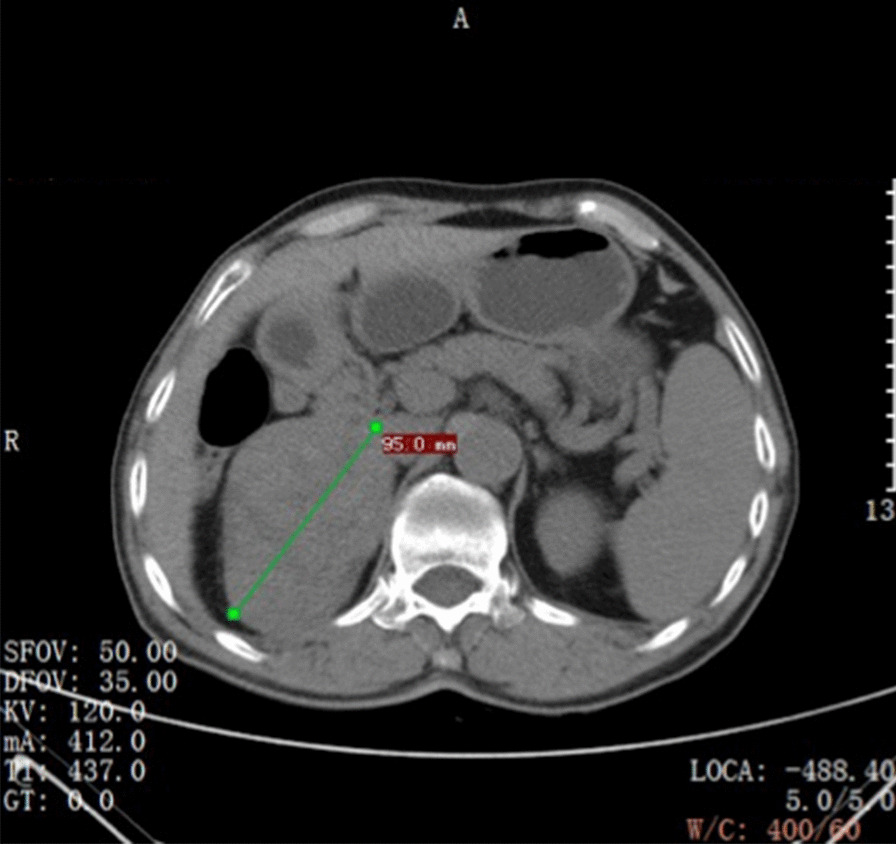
Non-contrast-enhanced CT scan showing an encapsulated large right adrenal lesion with regular margins
